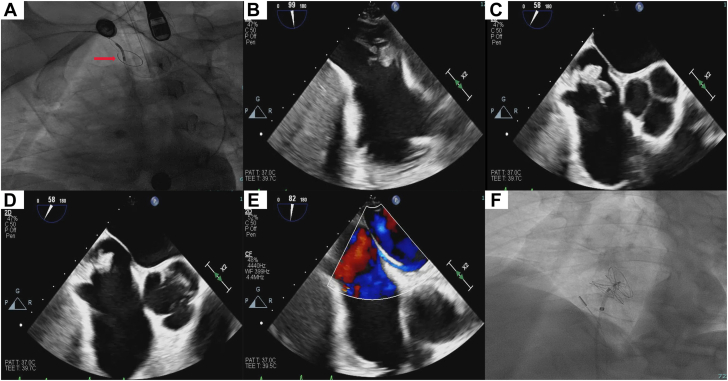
Periprocedural imaging. (A) Fluoroscopic view showing the SENTINEL device (red arrow). (B) Transesophageal echocardiogram (TEE) thrombus in contact with the FlowTriever. (C) TEE showing FlowTriever suctioning the thrombus. (D) TEE showing thrombus adherent to the tip of the FlowTriever device in a lollipop-like configuration. (E) TEE showing empty patent foramen ovale (PFO) with color Doppler flow across the PFO. (F) Fluoroscopic view showing PFO closure with 30.0-mm GORE CARDIOFORM Septal Occluder.
